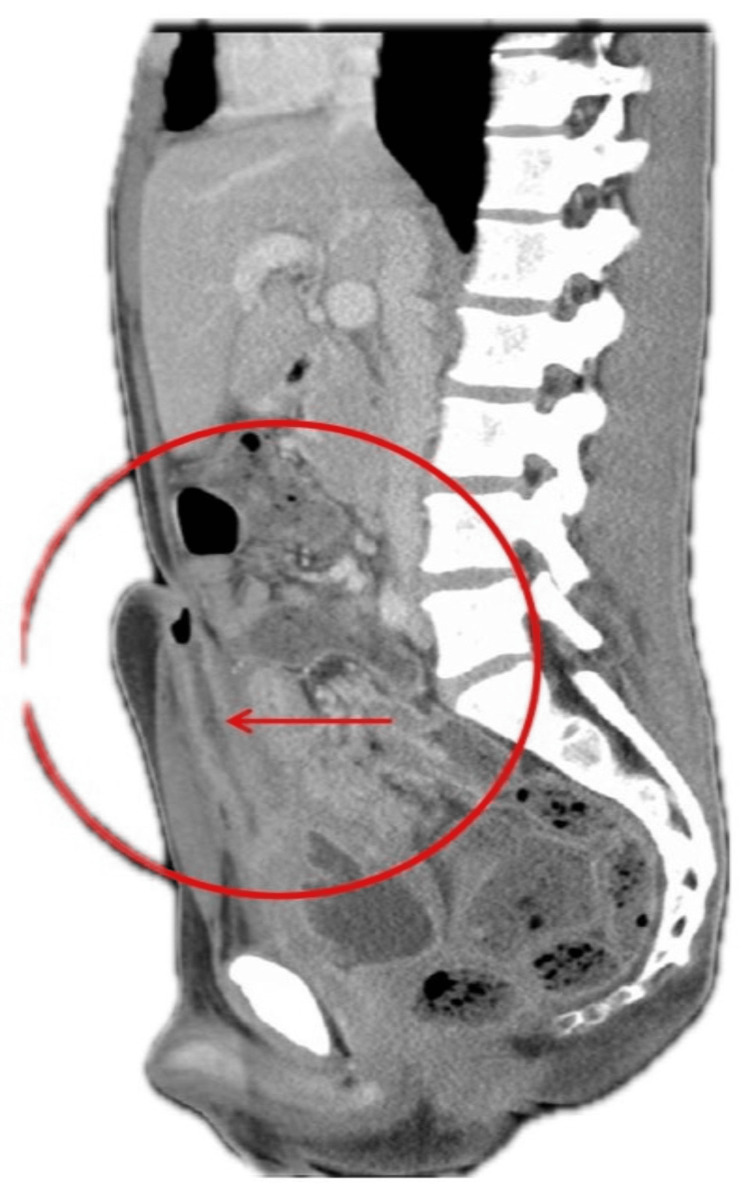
Sagital computed tomography showing fistulating tract (arrow) extending from the anterior wall of the bladder to the urachus remnant. Circle Marked inflammatory tissue of the small bowel adjacent to the superior aspect of the bladder.
